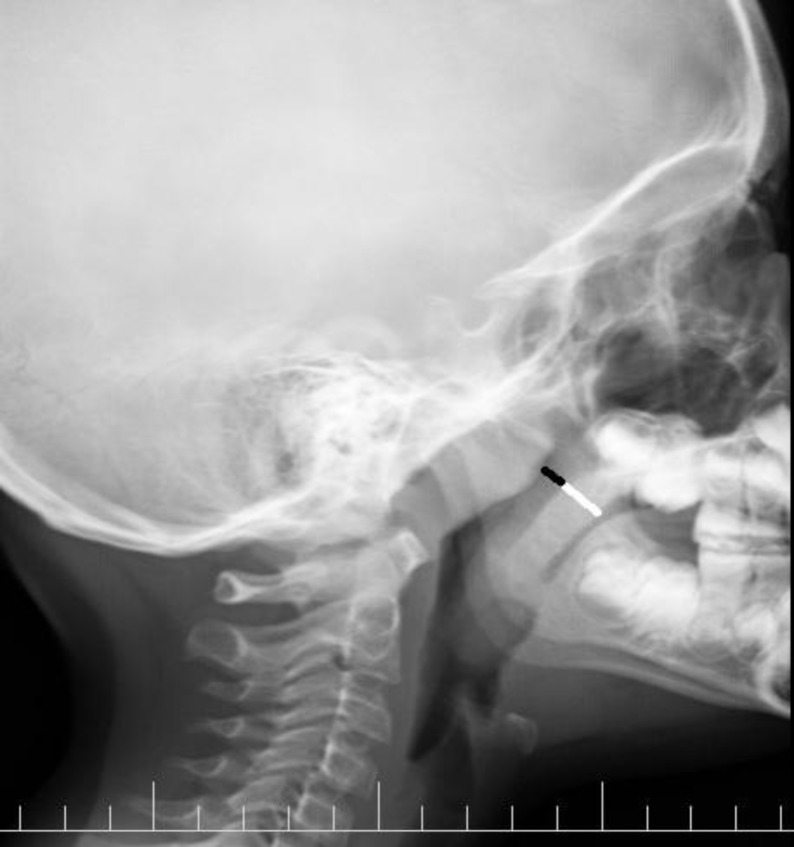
Airway (black line) to soft palate (white line) ratio method of assessing adenoid enlargement on lateral neck radiography as described by Cohen and Konak
